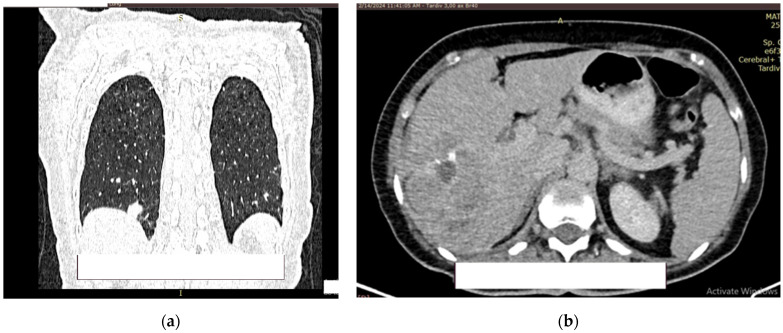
Contrast CT aspect. If there are multiple panels, they should be listed as: (a) Description of what is contained in the first panel; (b) Description of what is contained in the second panel. Figures should be placed in the main text near to the first time they are cited.
